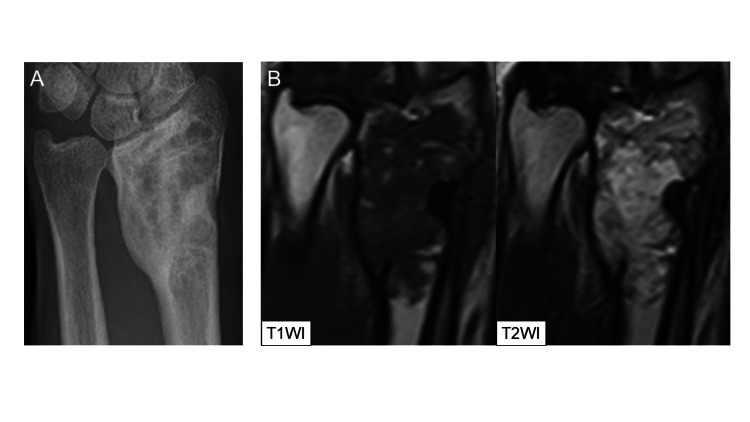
Images at one year after biopsy. A: The X-ray image showed further osteogenic features. B: The MRI images showed that the tumor borders became unclear and the tumor turned to a higher intensity on T2-weighted images.
